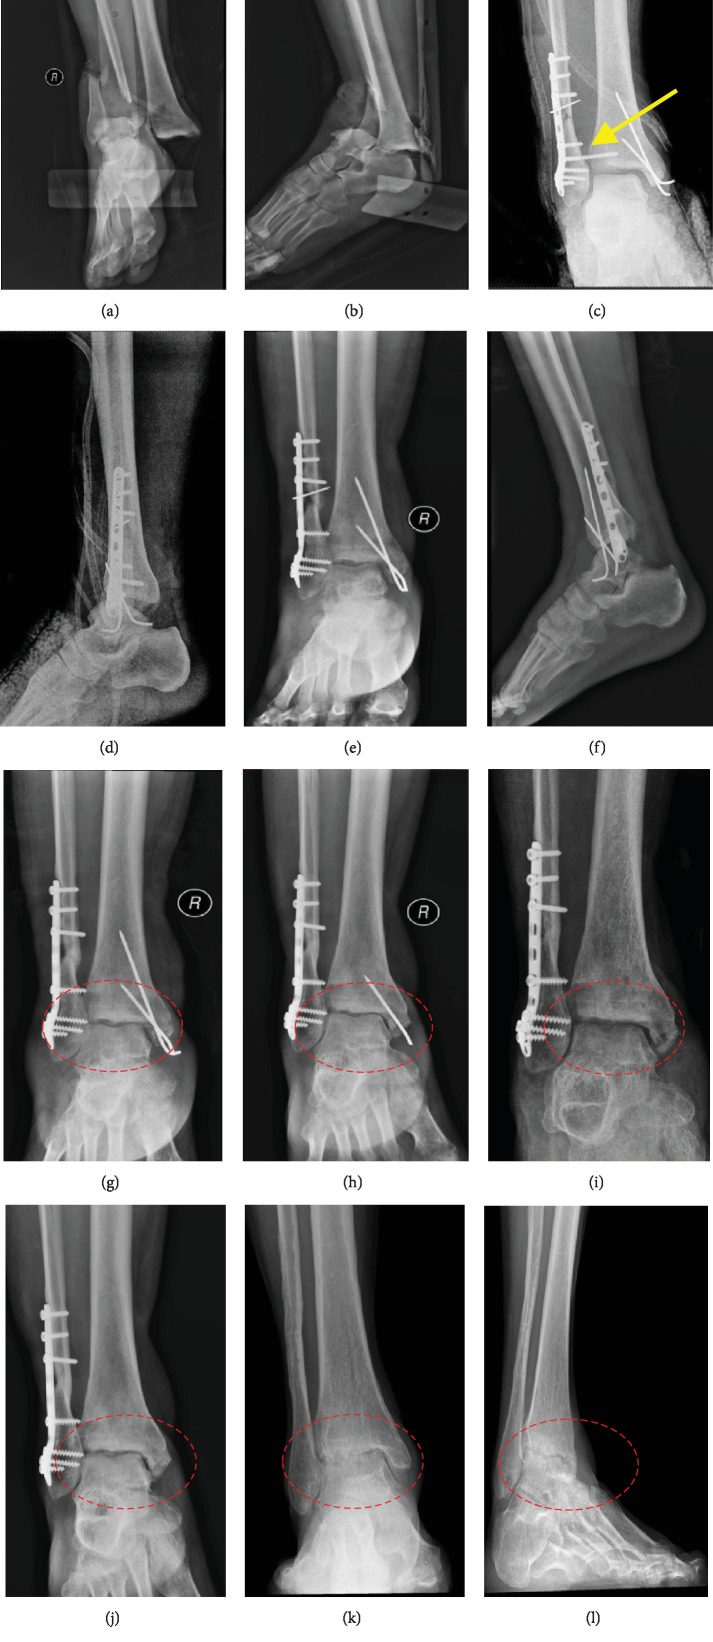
Preoperation (a, b) and postoperation radiographs after first reduction and debridement (c, d) from a 37-year-old man who suffered from falling from a height, showing the malreduction of the lower tibiofibular joint (yellow arrow). Thus, we performed revised surgery (e, f) to obtain acceptable anatomy of TFOL and TFCS. However, gradual degeneration of the tibiotalar joint and deterioration of the joint surface were observed along with the different follow-up (red circle with dot line) (g–j). Finally, significant posttraumatic osteoarthritis occurred at the ankle joint at the last follow-up (k, l).
